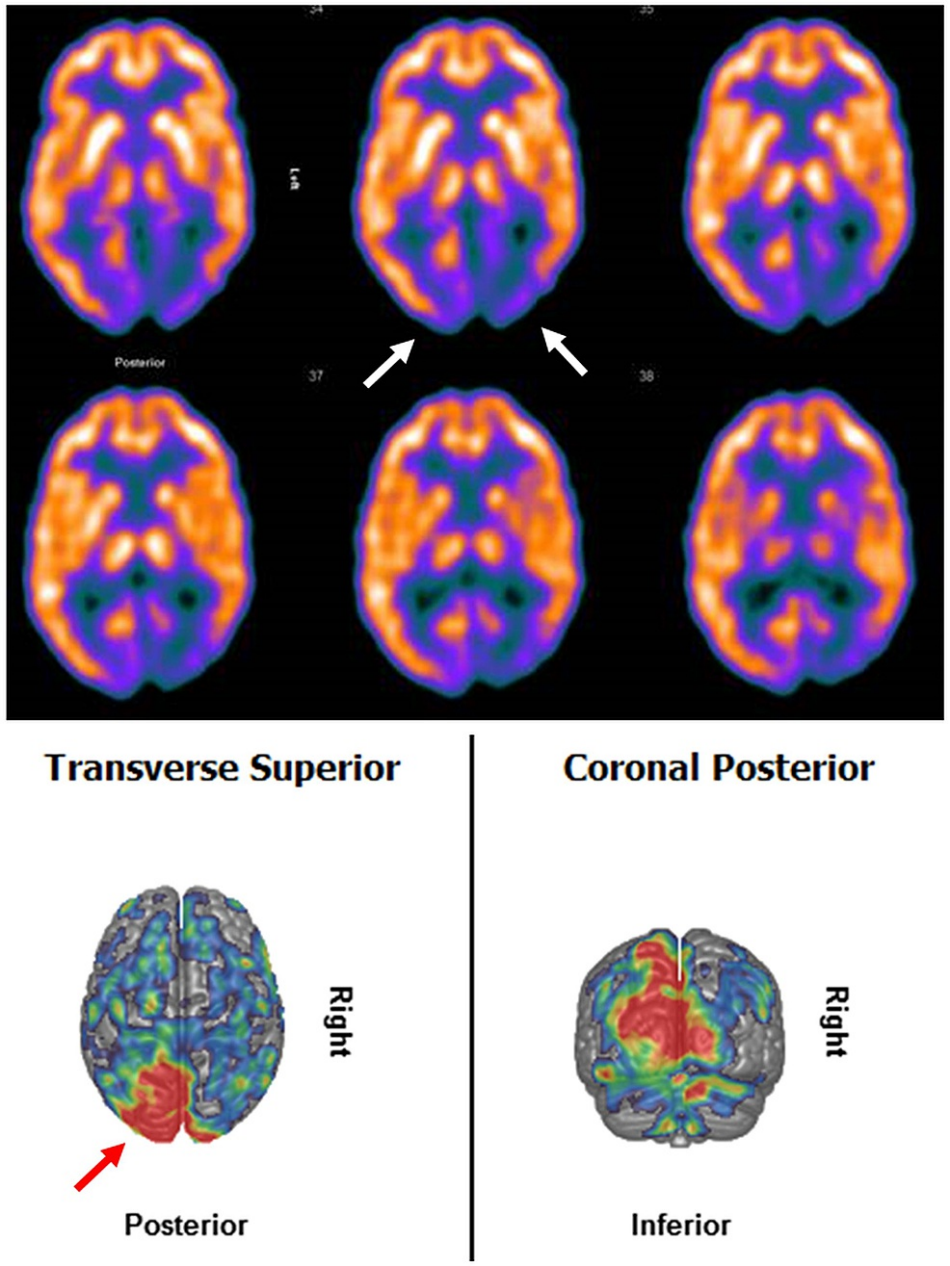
Occipital hypometabolism on 18F-FDG PET (301 MBq 18F-FDG, Siemens Exact, Knoxville, USA) with left-sided predominance during the second SLE at age 49 years. Upper panel: trans-axial slices with violet areas indicating hypometabolic occipital cortex. Lower panel: 3D cortical surface compared to an age-matched normal collective, red areas indicating significant hypometabolism in the cerebral cortex.
